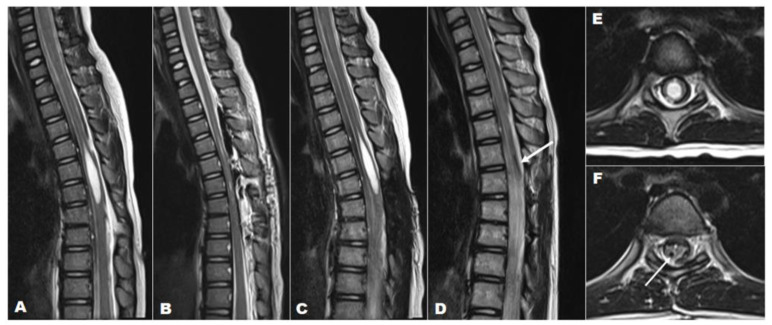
A 7-year-old girl with a large loculated syringomyelia, T4–T6, caused by a dermal sinus tract. Serial sagittal MRI images (T2-weighted) demonstrate (A) the preoperative syrinx (T4–T6) with TCS, (B) the complete syrinx resolution immediately post-untethering and simple drainage, (C) the syrinx recurrence at the previous location at 6 months post-untethering with almost the same size as the preoperative syrinx, and (D) the absence of the syrinx and the proximal catheter that was previously implanted (white arrow) at follow-up after 6 years post-shunt surgery. T2-weighted axial MRI indicating (E) the preoperative syrinx (SI > 0.8) and (F) the complete resolution of the syrinx and the proximal catheter (white arrow) at final follow-up 6 years after shunt surgery.
